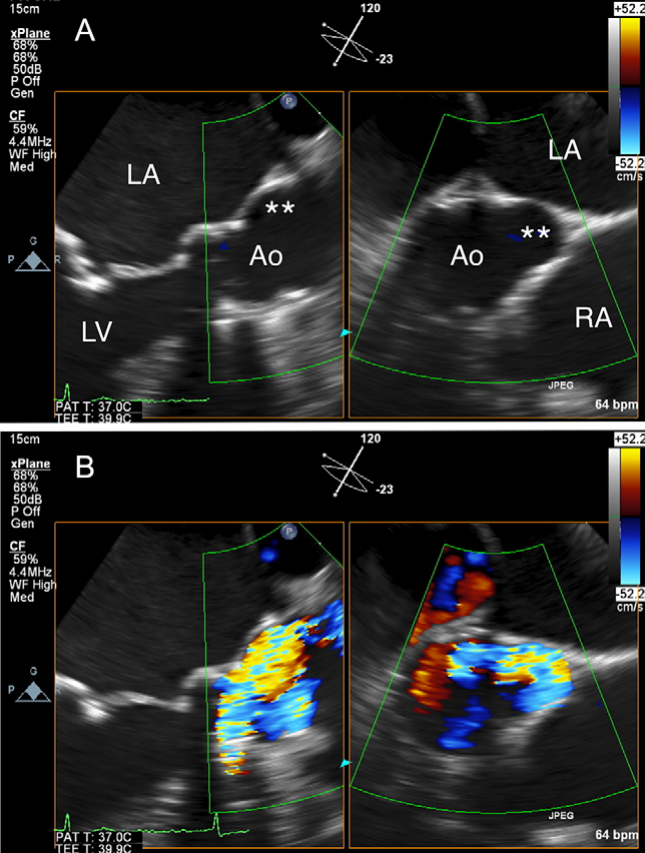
(A) Transesophageal echocardiography (TEE) revealed a saccular ascending aortic aneurysm arising from the posterior ascending aortic wall just above the sino-tubular junction. (B) TEE with color Doppler revealed eccentric systolic flow jets through the tilting disc valve prosthesis directly impinging on the saccular aneurysm. Ao: aorta; LA: left atrium; LV: left ventricle, RA: right atrium; Double asterisk: saccular aneurysm.
